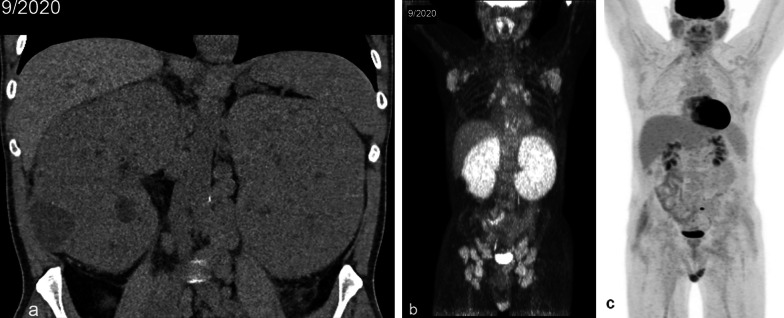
Nephromegaly of renal lymphoma*. Coronal noncontrast CT (a) demonstrates symmetric marked enlargement of the bilateral kidneys from lymphomatous infiltration in this patient with mantle cell lymphoma. Concurrent PET/CT in 9/2020 (b) demonstrates marked F18-FDG avid bilaterally enlarged kidneys as well as extensive supradiaphragmatic and infradiaphragmatic lymphadenopathy. Follow-up F18-FDG PET/CT in 11/2020 (c) following chemotherapy shows complete metabolic and radiologic response with normalized renal size and activity
